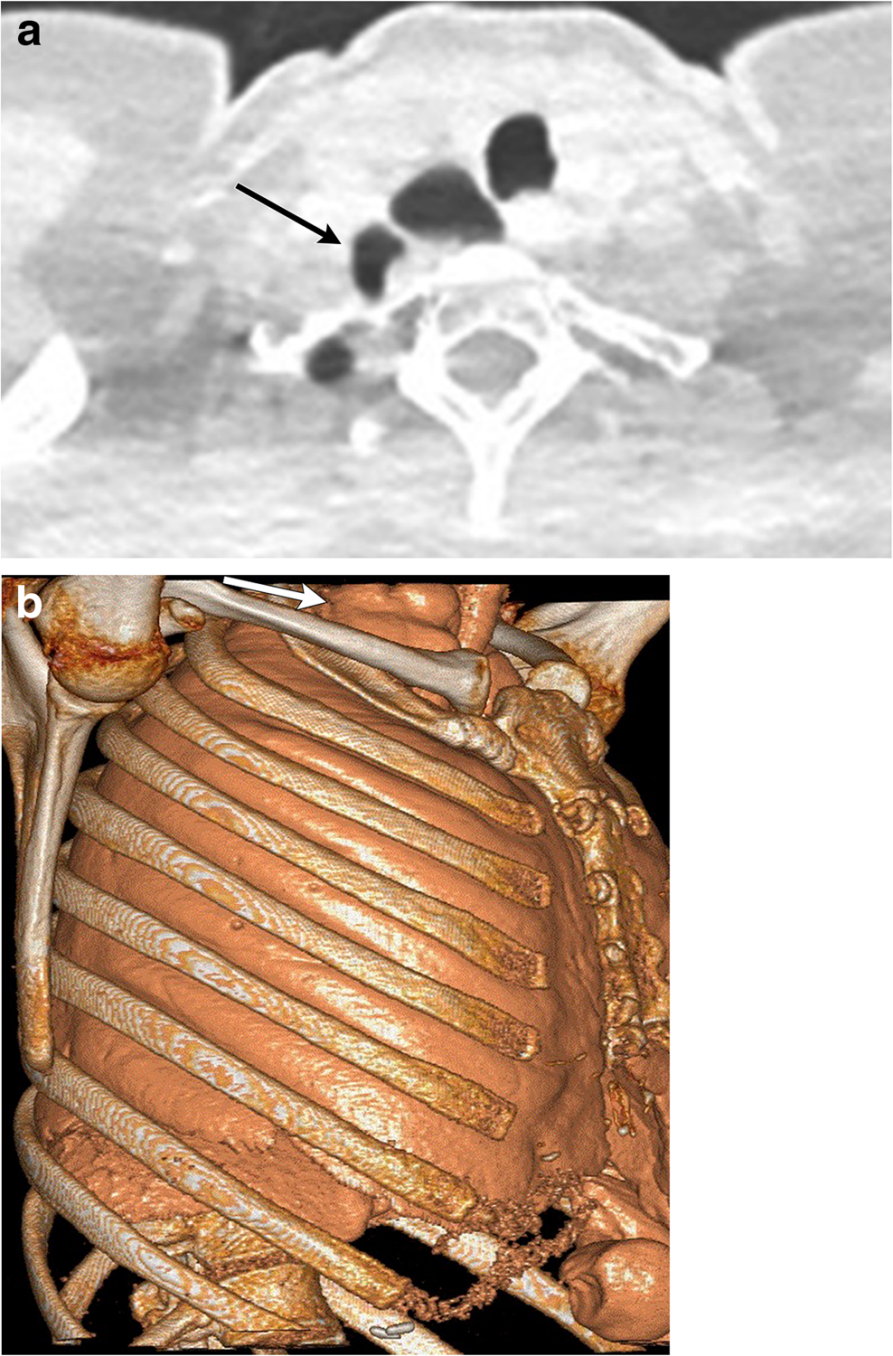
a, b A 67-year-old female being evaluated for pulmonary nodules and recent acute exacerbation of reactive airway disease. Axial CT (a) demonstrates the apicoposterior segment of right upper lobe (black arrow) extending into the cervical region. Volume-rendered image (b) clearly depicts the herniated lung above the clavicle and first rib (white arrow). Herniated lung causes a smooth impression on the trachea. These can cause tracheal deviation or compression
